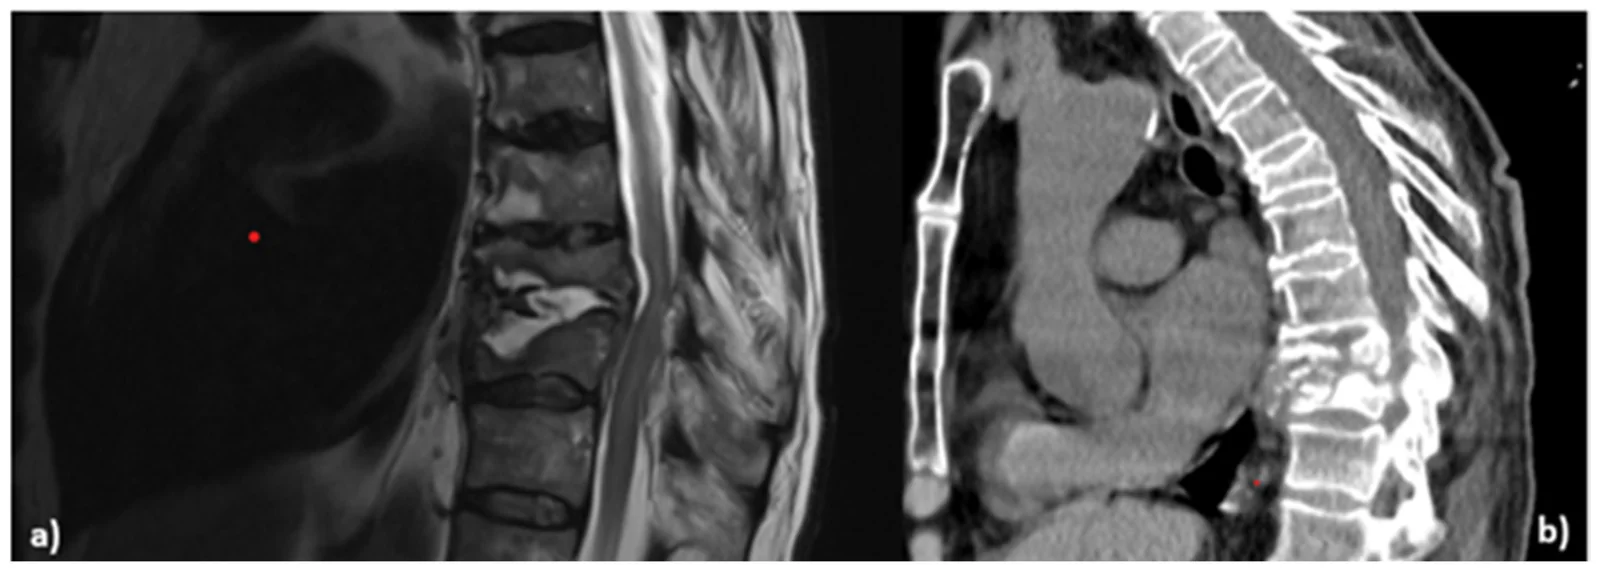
Pyogenic spondylodiscitis on MRI and CT. Sagittal T2-weighted MRI (a) and sagittal CT (b) demonstrate T9–T10-centered destruction of the disc–endplate complex, with marked disc-space involvement, irregular erosion of the opposing endplates, and ill-defined paravertebral and epidural inflammatory extension causing spinal canal narrowing and cord compression. The disc-centered destructive pattern and phlegmonous soft-tissue extension favor PS over TS. Vertebral collapse alone is not discriminatory, as it may also occur in advanced TS.
